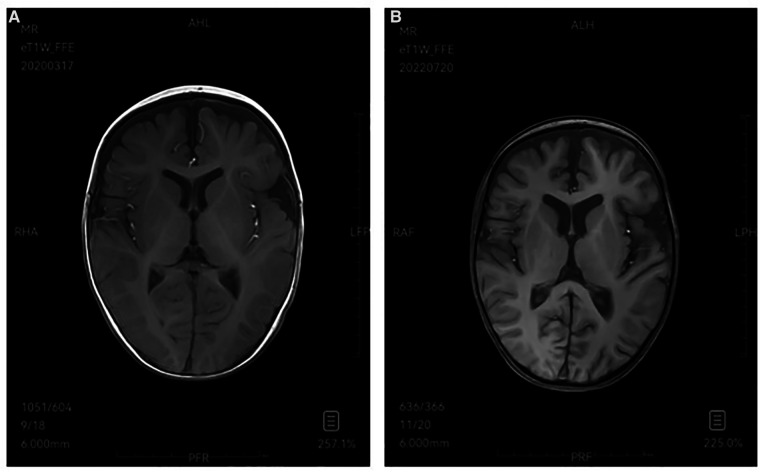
(A) 2020-3 MRI-T1W image demonstrates bilateral frontotemporal extracerebral space widening. (B) 2022-7 MRI-T1W image shows brain atrophy.
